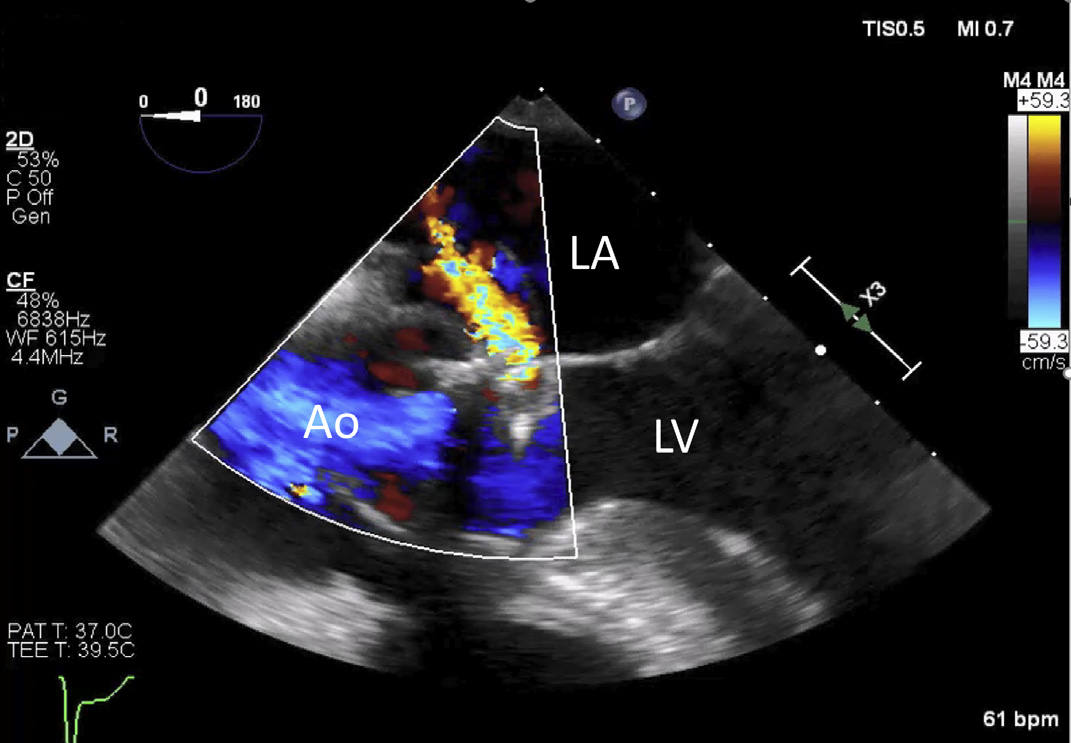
Transesophageal Echocardiography With Color Doppler Showing Left Ventricular Outflow Tract-to-LA Fistula Ao =aorta; LA = left atrium; LV = left ventricle.
